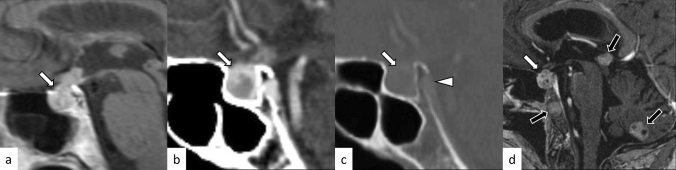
(a–c, arrows) A 76-year-old woman with pituitary metastasis of breast cancer. Post-contrast T1-weighted sagittal image shows heterogeneously enhanced mass in the pituitary gland and stalk (a). Contrast-enhanced CT shows the mass extending posteriorly through the clivus with evidence of bone destruction (b, c, arrowhead). (d, arrow) A 60-year-old woman with pituitary metastasis of small cell lung cancer. There are multiple other metastatic lesions involving the pineal region, cerebellum, and clivus (d, black arrows)
